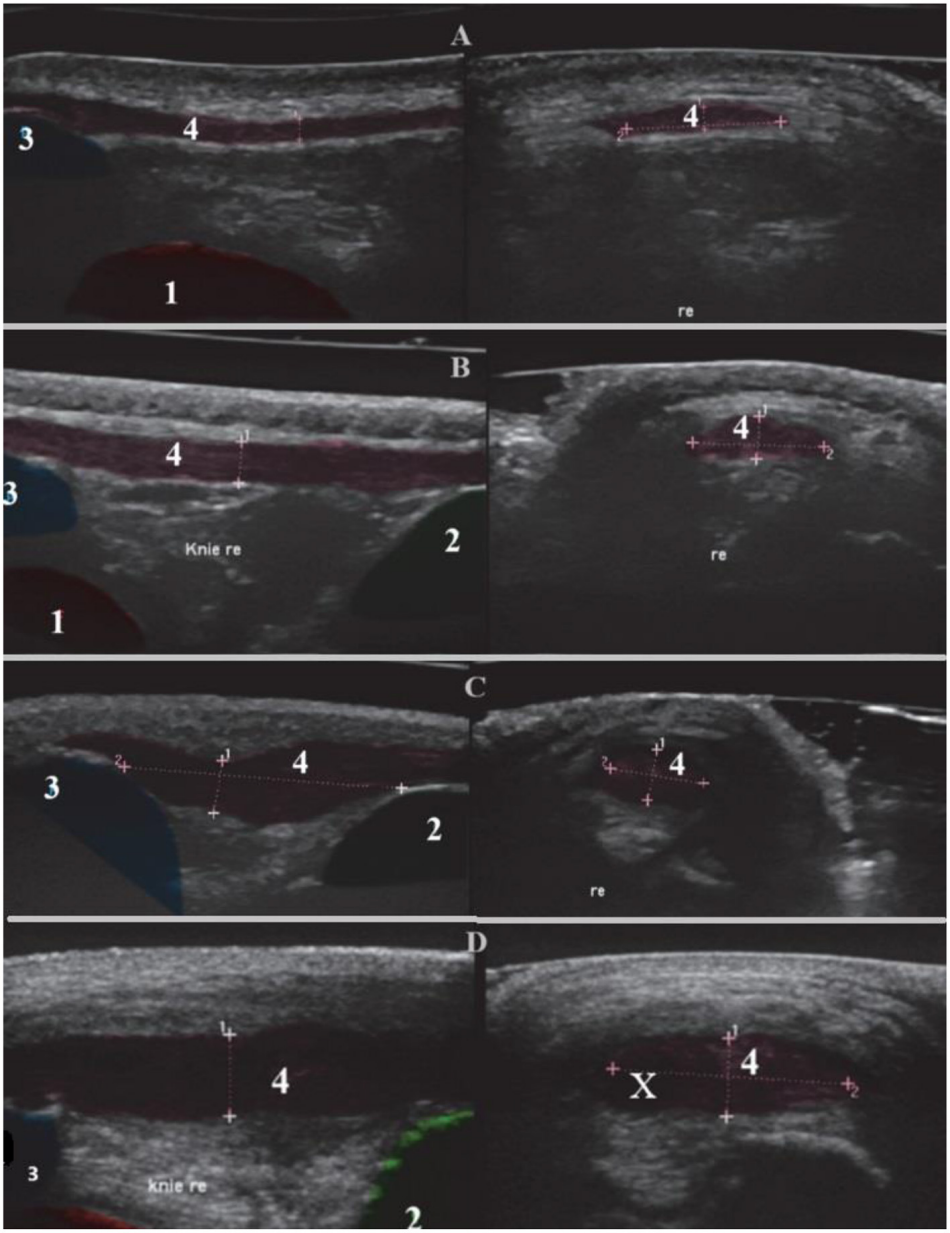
Sonographic representation of the right patellar ligament with changes. Sagittal section (left), transverse section (right). (1) Femur, (2) Tibia, (3) Patella, (4) Patellar ligament with fiber alignment, *Re* right and (X) Core lesion. (A) Grade 0, Boxer, 8 years old, female. (B) Grade I, Golden Retriever, 6 years old, female. (C) Grade II, German Shepherd, 9 years old, male. (D) Grade III, Mixed breed, 5 years old, male.
